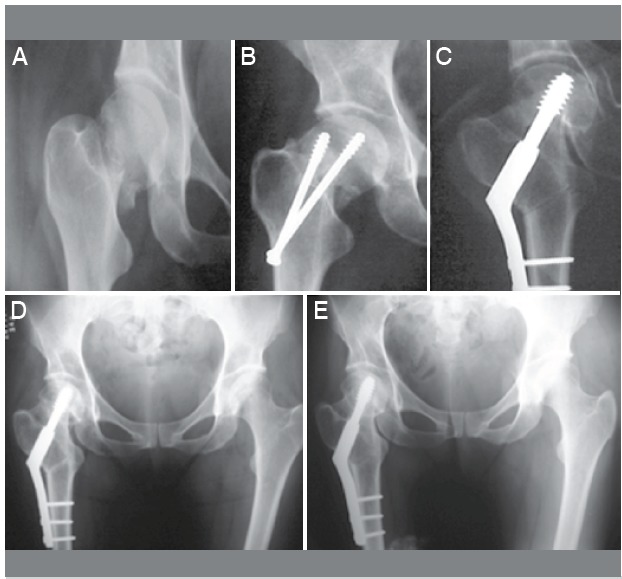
Femoral neck fracture (A); (B) failure of initial osteosynthesis treat-ment with two cannulated screws; (C) post-operative valgus intertrochanteric osteotomy; (D) and (E) pelvis x-ray showing the consolidation of valgus os-teotomy and healing of non-union neck fracture in one year and 7 years later.
